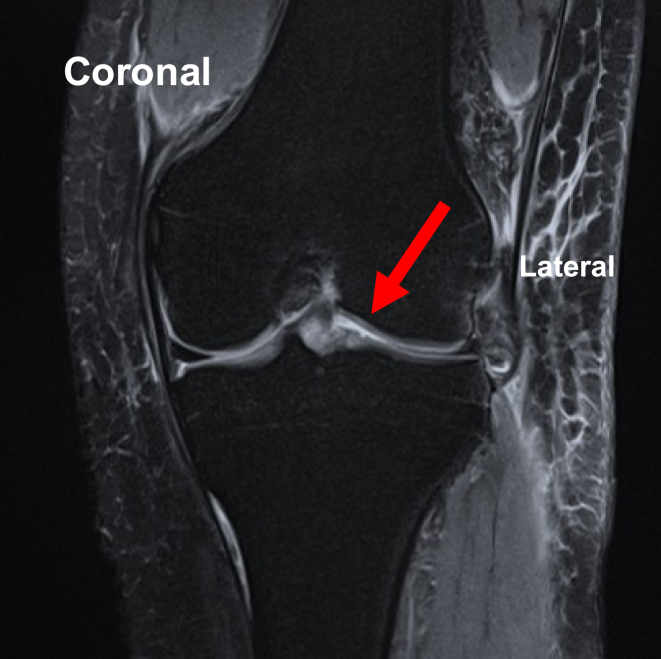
Postoperative coronal magnetic resonance imaging showing the reduction of the lateral meniscus tear (arrow) in the left knee.
